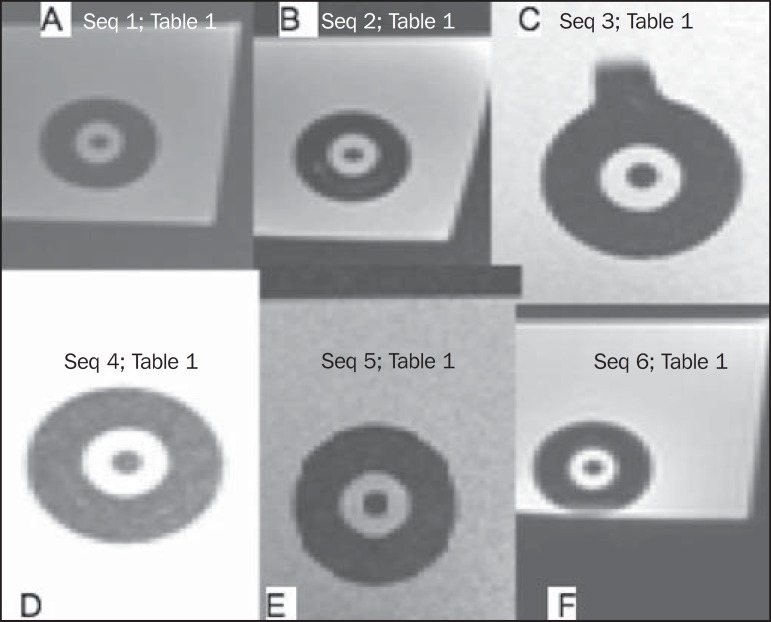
MRI scan (T2-weighted sequence) in which the CuSO_4_, ring lumen
